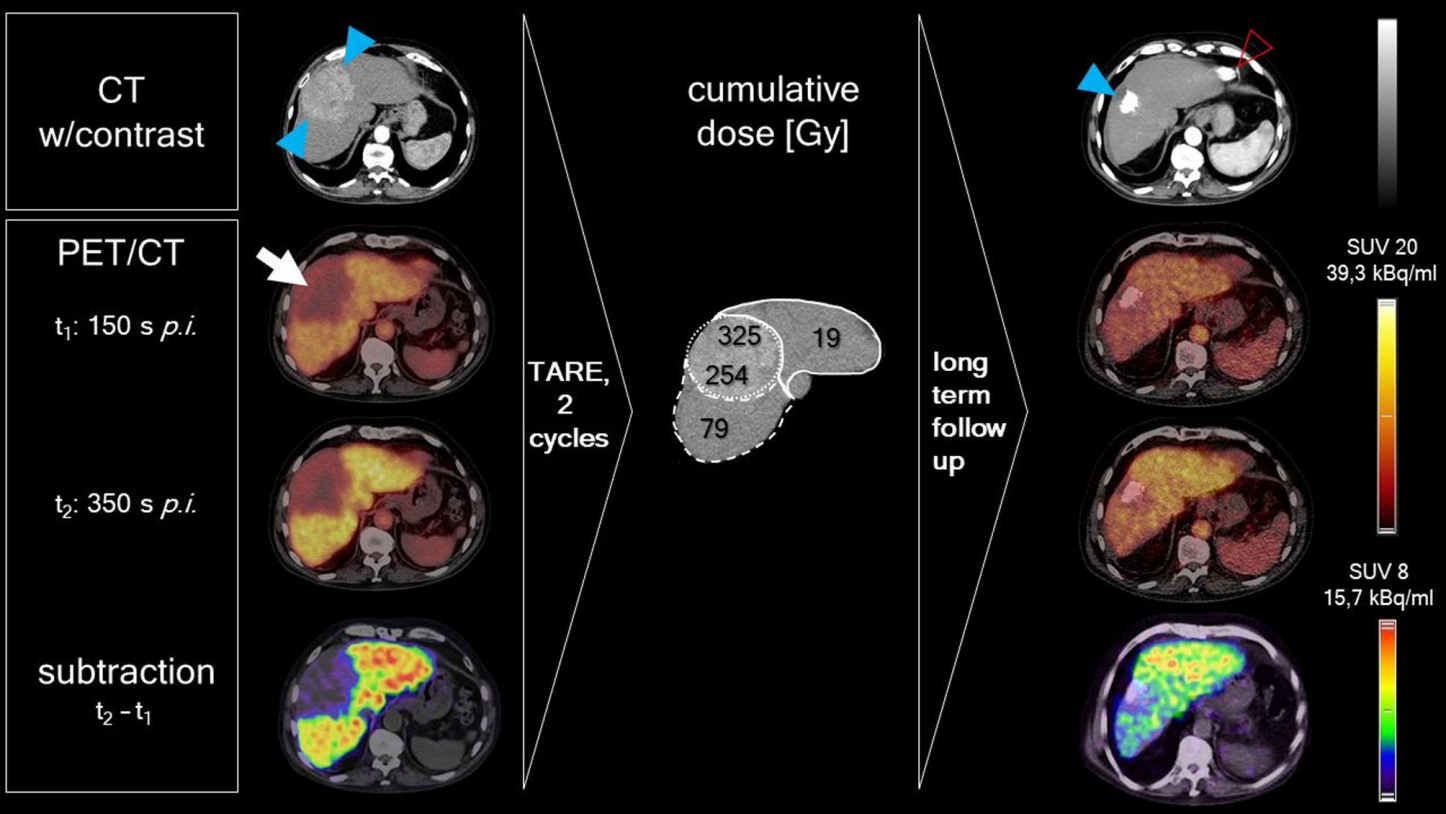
The solitary HCC of the 82-year-old patient involved both liver lobes. Contrast-enhanced CT (upper row) and [^68^Ga]Ga-BP-IDA-PET/CT (second row 150 s *p.i.*; third row 350 s *p.i.*) were performed before and after two TARE treatments. The hypervascular HCC (blue arrowheads) is seen as an area of low tracer uptake (white arrow). Subtraction of the early (150 s *p.i.*) PET dataset from the late (350 s *p.i.*) PET dataset gave a subtraction image visually highlighting the increase in activity concentration (bottom row). TARE treatment of the left and right liver achieved complete remission of the tumor with hyperdense areas corresponding to previous microsphere accumulations (blue arrowhead). The cumulative energy doses in [Gy] in the tumor area and in the non-targeted lobes are denoted by black numbers in the middle. On the CT after TARE, the heart is visible (red arrowhead)
